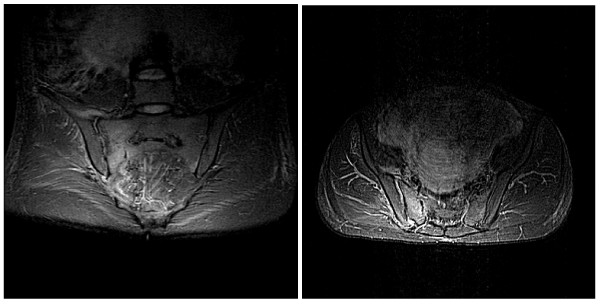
Coronal and axial short inversion-time inversion-recovery sequence magnetic resonance imaging of the sacrum shows the fracture line of the right lateral mass surrounded by an area of edema.
